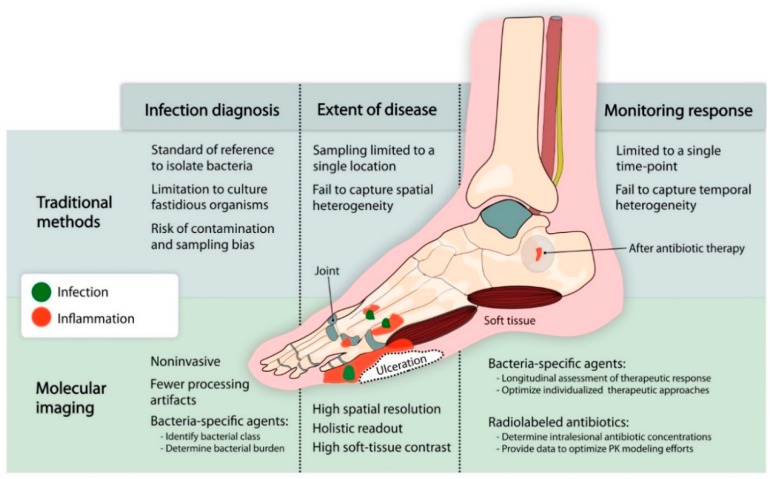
Limitations of traditional methods and opportunities for molecular imaging to diagnose and monitor diabetic foot infection (DFI). Diabetic foot infections have traditionally been evaluated using invasive techniques (e.g., microbiological cultures, bone biopsy) that can miss the site of infection and do not reflect the heterogeneity of the disease, or by non-specific methods (e.g., serum biomarkers, plain radiographs, etc.) that cannot correctly differentiate between infection and sterile inflammation (e.g., Charcot’s neuroarthropathy). Novel developments in molecular imaging provide new non-invasive tools that can accurately differentiate infection from inflammation, determine the location and extent of the disease, characterize the conditions of the microenvironment at the infection site, and monitor the response to antibiotic therapy.
